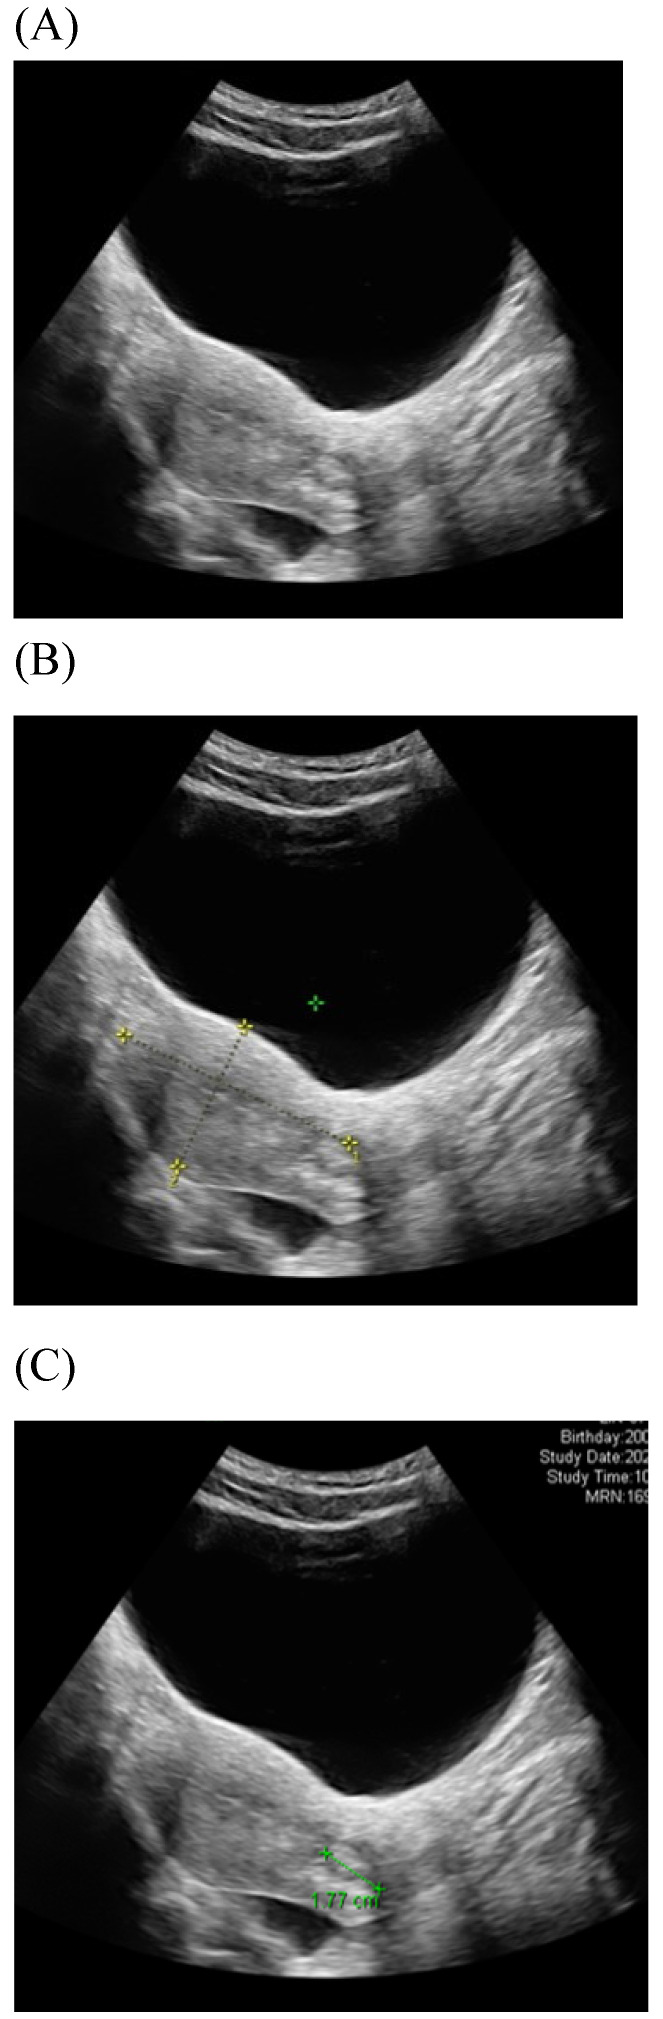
Transabdominal ultrasound image shows a longitudinal section of the uterus with normal cervix of the female aged17-year-old. (A) Sagittal view (B) uterine size (C) cervical length.
